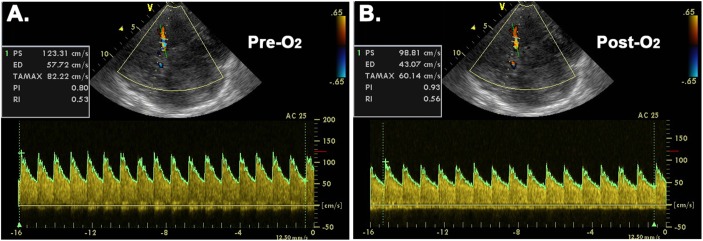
Representative transcranial color-coded duplex sonographic images before (A) and after (B) O_2_-inhalation. Pre-O_2_ = before O_2_-inhalation; Post-O_2_ = after O_2_-inhalation; AC = angle correction; PS = peak systolic velocity; ED = end diastolic velocity; TAMAX = time-averaged maximum velocity; PI = pulsatility index; RI = resistive index.
